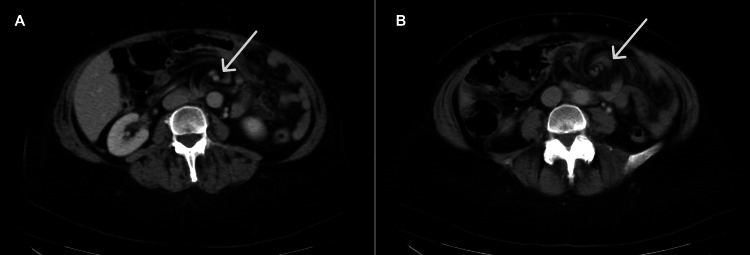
CT abdomen and pelvis (axial). The mesenteric swirl sign, indicative of mesenteric edema and distortion of vessels and bowel loops, can be seen at the arrow in two slices (A: superior; B: inferior)
